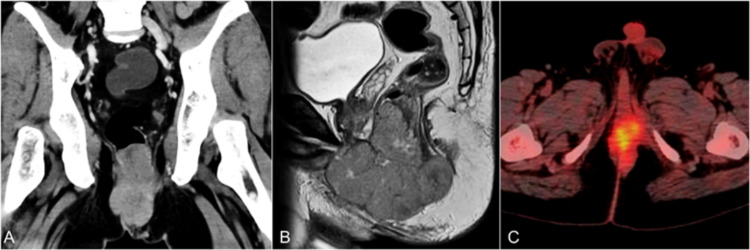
Pretreatment cross-sectional imaging (A) Coronal reconstruction of CECT showing a heterogeneously enhancing soft-tissue density lesion measuring 9.1 x 5.4 x 7.8 cm involving the anal canal with loss of fat plane with levator ani bilaterally. (B) Sagittal reconstruction of T2 MRI showing a large lobulated lesion involving the anal canal with extension into the perineum and anorectal junction. (C) FDG-PET CT fusion image showing metabolically active disease in the anal canal. CECT: contrast-enhanced computed tomography, MRI: magnetic resonance imaging, FDG-PET: fluorodeoxyglucose positron emission tomography.
